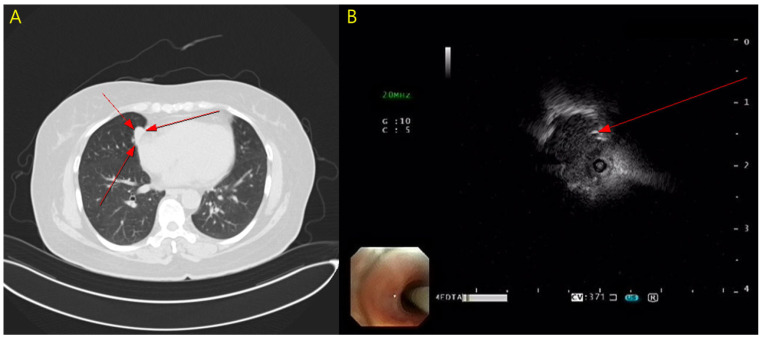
Representative case of successful RP-EBUS localization in a pulmonary nodule without CT-BS: (A) Chest CT demonstrating a pulmonary nodule (arrow) without an identifiable CT bronchus sign. (B) RP-EBUS image showing a dense sign with an adjacent orientation relative to the target lesion (arrow). RP-EBUS, radial probe endobronchial ultrasound-guided; CT-BS, computed tomography bronchus sign.
